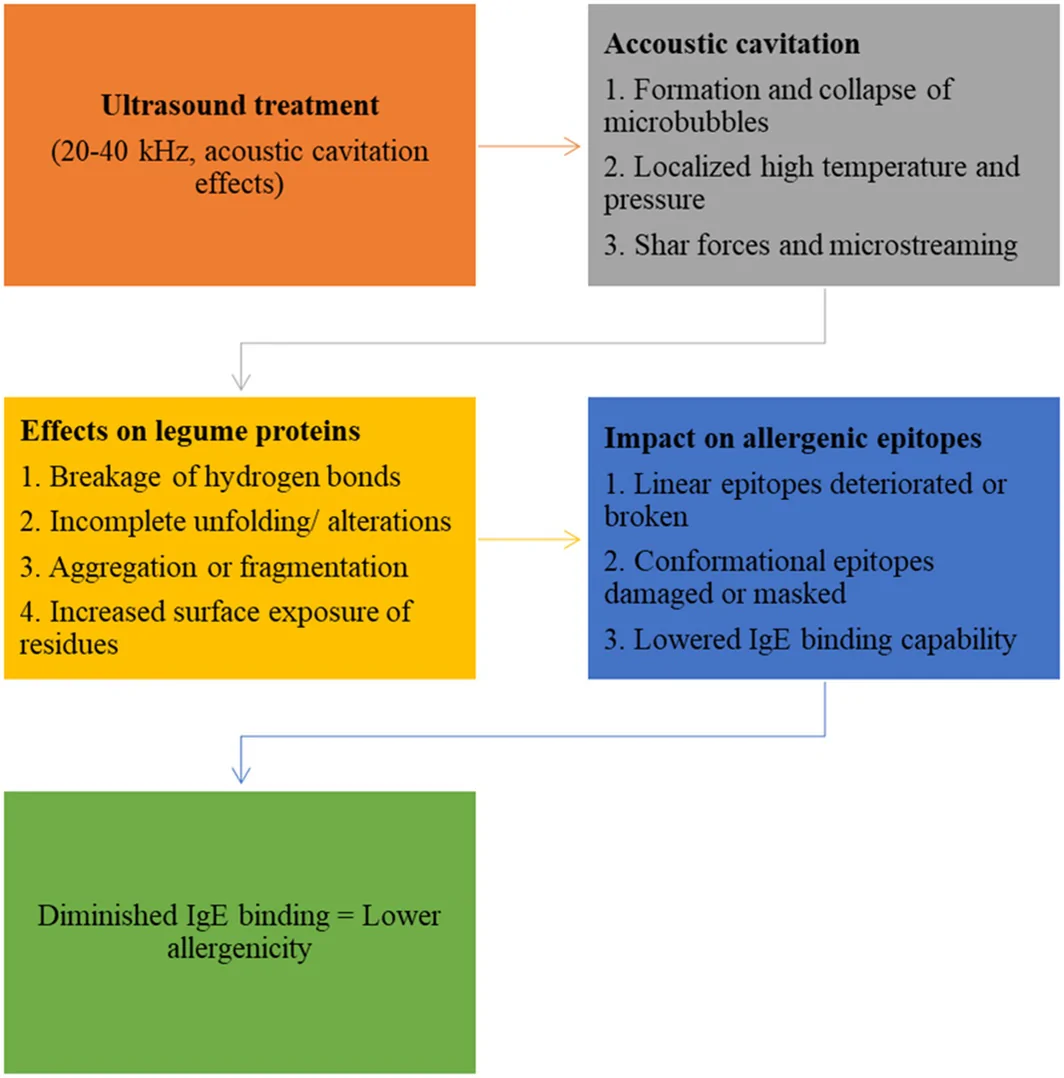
Mechanisms of ultrasound (US) in legume allergenicity reduction (adapted from Yang et al. 2015).
